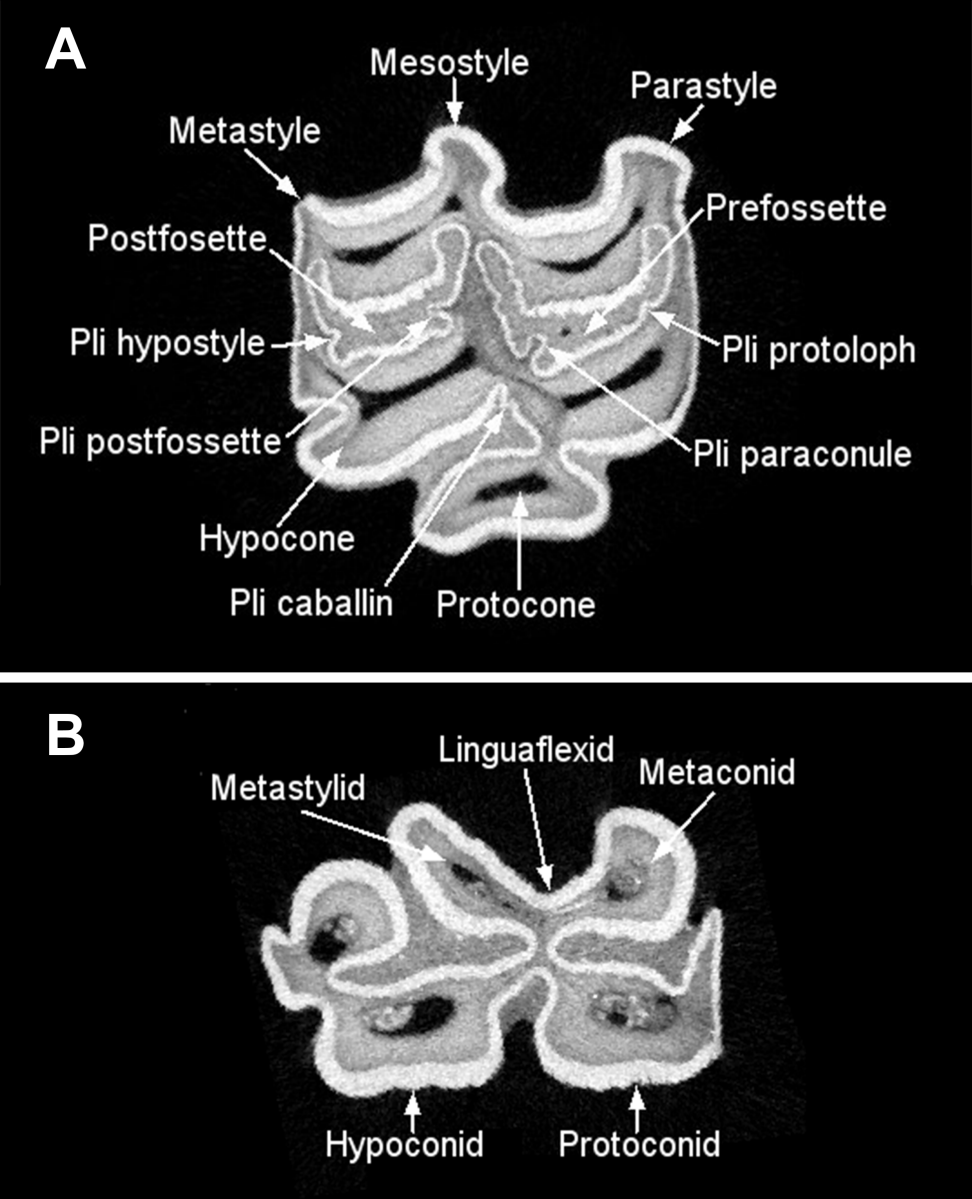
Upper (A) and lower (B) fourth premolars showing the dental structures referred to in the text, following the dental nomenclature of Evander [64]. Computed tomography (CT-scan) images of LACM 192/156497 (A) and TMM 937–169 (B). The anterior side of both teeth is located to the right. The lingual side is located at the bottom in figure A and the top in figure B.
